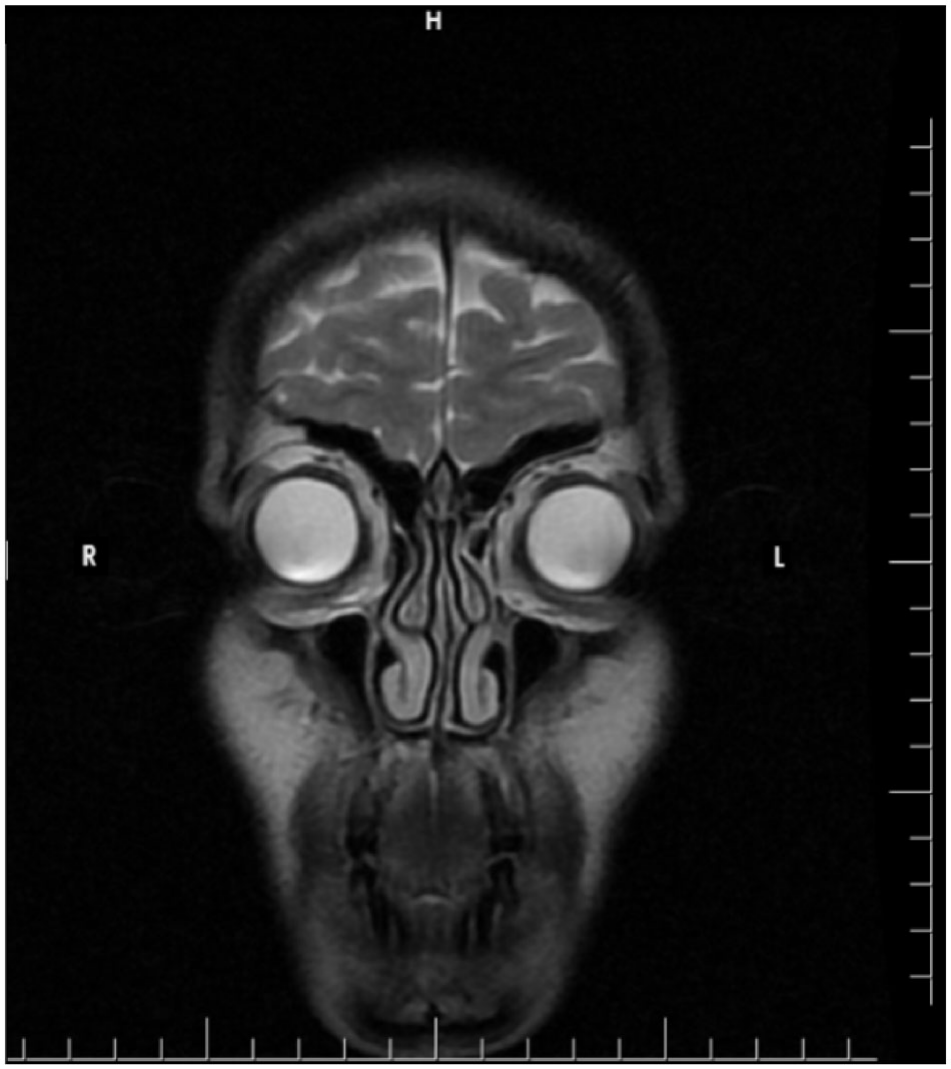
The MR image of the patient taken before the application. MR imaging shows that there are no pathology in bilateral frontal, ethmoid and maxillary sinuses.
